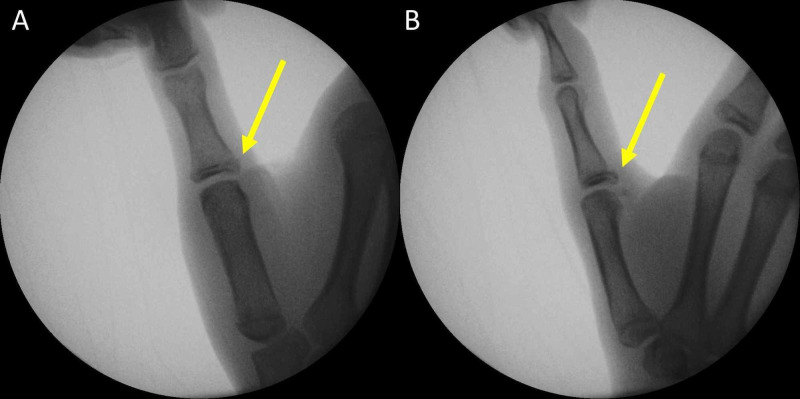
X-rays of the thumb after completion of surgery Anatomic reduction of the avulsed attachment of the UCL is seen on the anteroposterior (A) and lateral (B) x-ray views of the operated thumb. The arrows indicate the location of the fracture. UCL: ulnar collateral ligament
